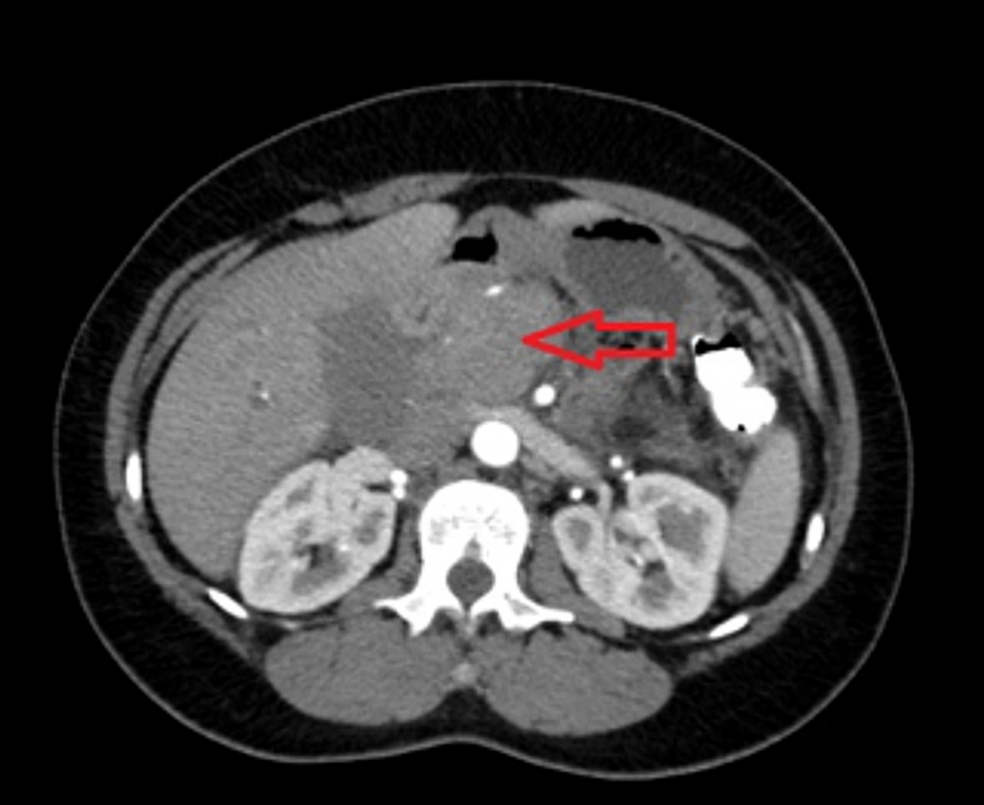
Pre-steroid treatment CT angiogram of the chest and abdomen revealing enlarged pancreatic head/uncinate process (red arrow) along with soft tissue density extending inferiorly and measuring 4.0 × 3.7 cm. CT: computed tomography
